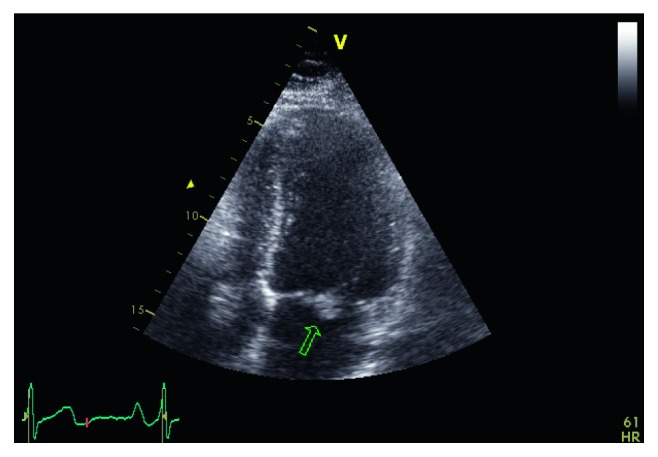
Echocardiogram showing ruptured mitral chordae tendineae.
